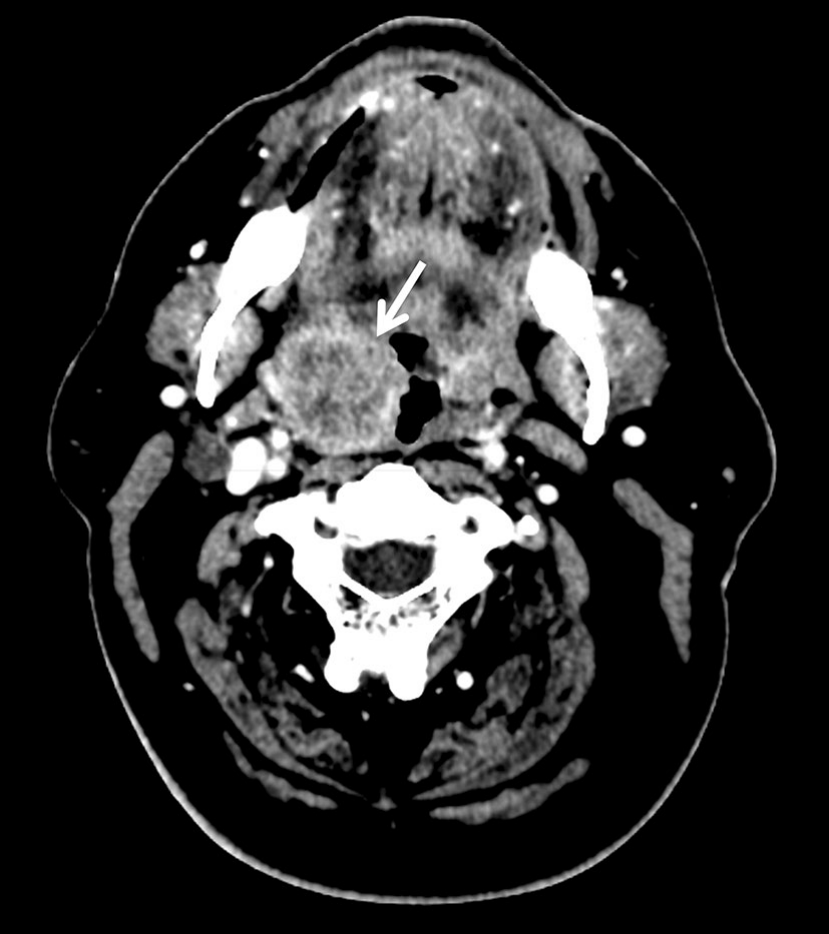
Axial CT slice showing well-defined contours of an exophytic P16+ tumour of the right palatine tonsil (arrow) in a 57-year-old male non-smoker.
